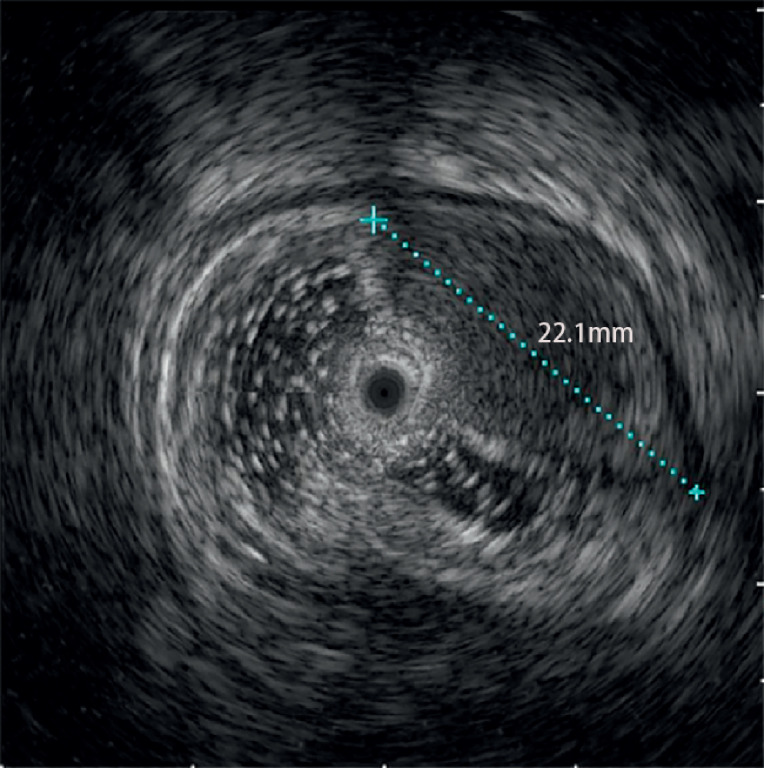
Endoscopic ultrasonography showing a 2.2-cm submucosal lesion.
